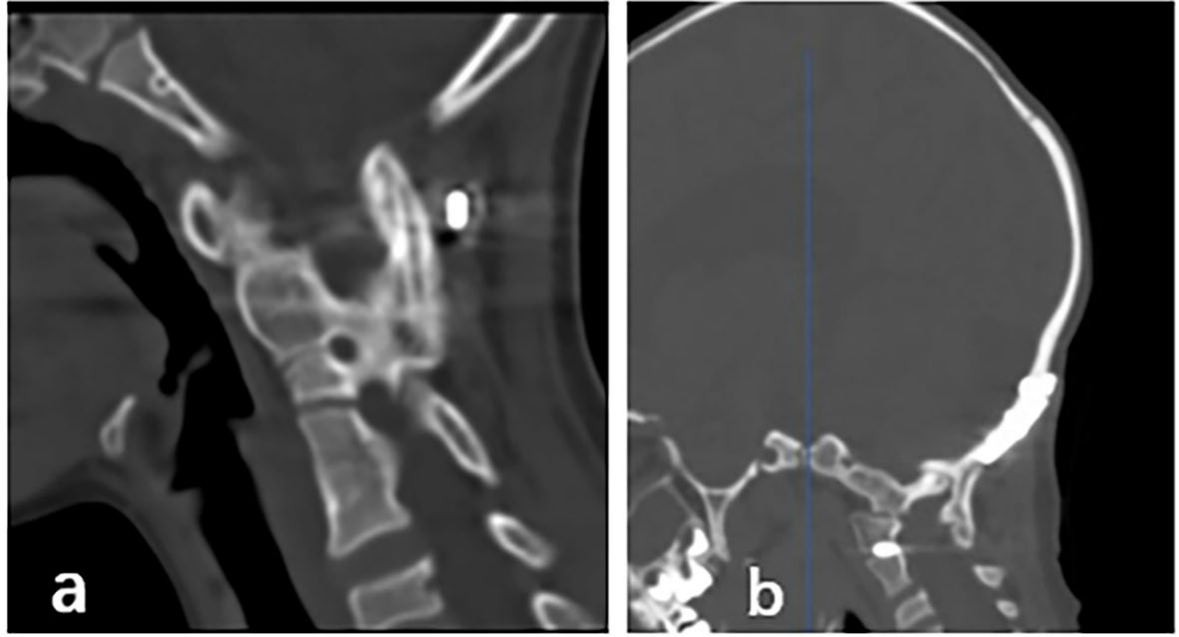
a, b: CT images of 2 children with fusion in the craniocervical junction area at 12 months and 18 months postoperatively, respectively, showed solid implant fusion in the region of the graft
